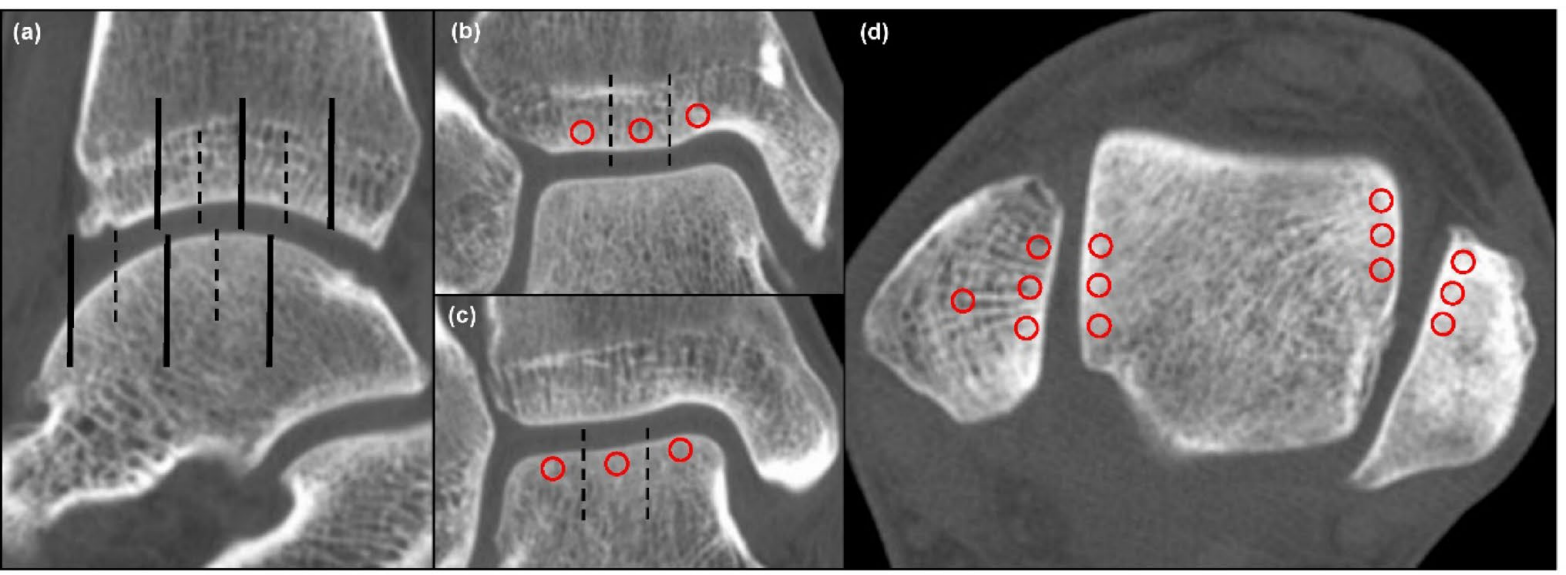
Measurement of the Hounsfield Unit values. a Scan lines in the sagittal slice of computed tomography. Dashed lines indicate the borders of the anterior, middle, and posterior parts. Black lines indicate scan lines of the tibia and talus, respectively. Three regions of interest (ROIs) (red circles) were set at the subchondral bone in the tibial plafond (b) and talar dome (c) in each coronal slice. Dashed lines indicate the borders of the medial, central, and lateral parts. d ROIs (red circles) of the medial and lateral gutters, and the center of the fibula as a reference
